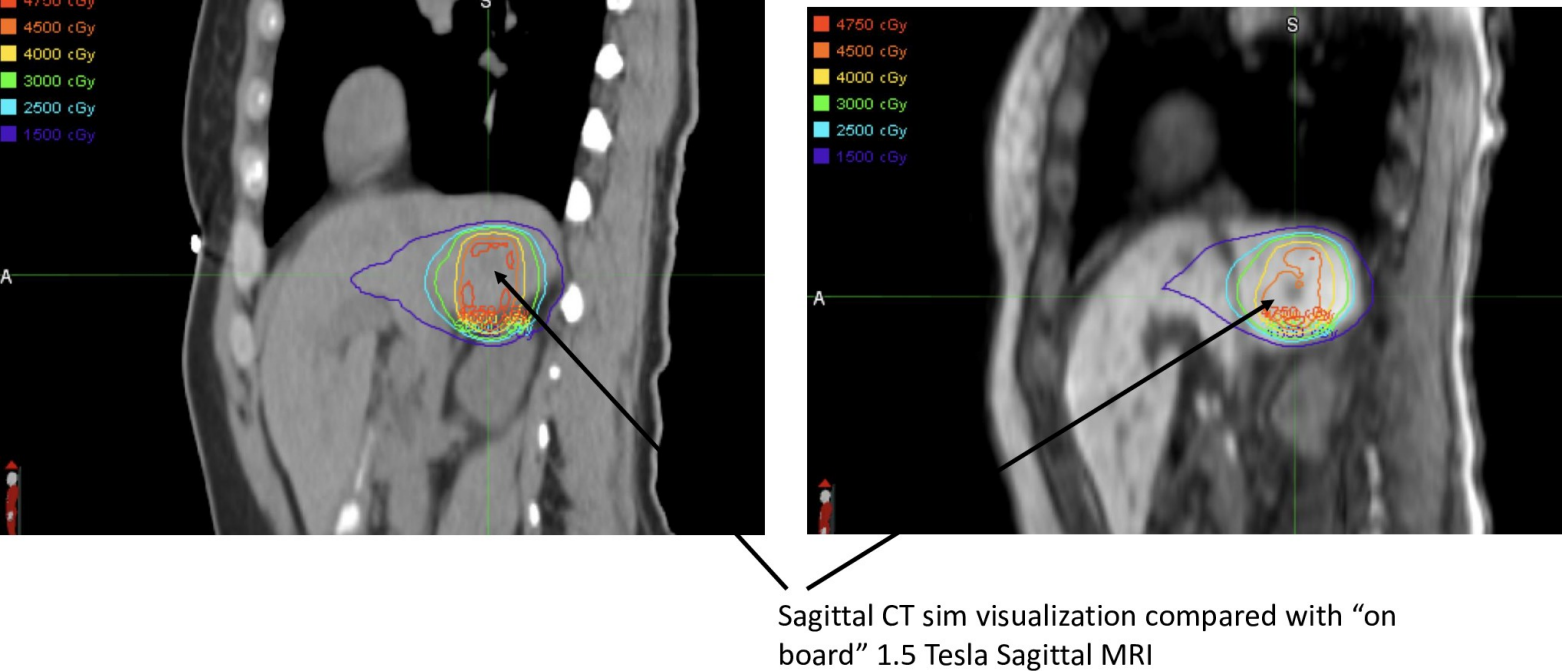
Improved visualization with 1.5 Tesla MRI as compared with CT on rails.
